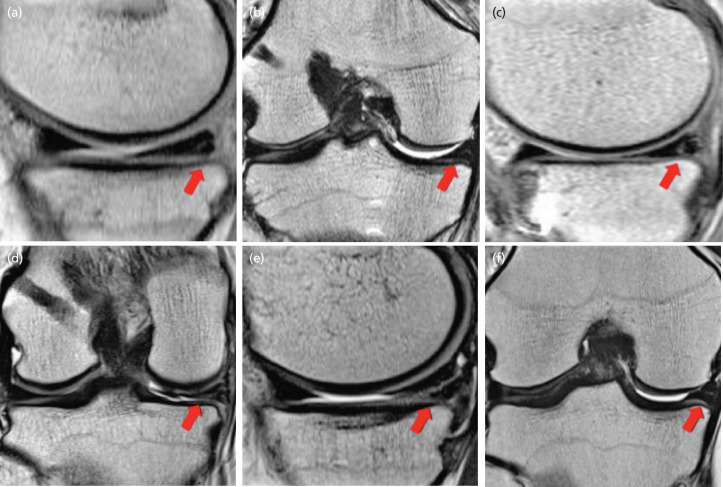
Crues’ grading system using magnetic resonance imaging (a, b) Grade 1 in sagittal and coronal view. No abnormal signal is seen on the meniscus. (c, d) Grade 2 in sagittal and coronal view. Red arrows indicate linear signal without communication with an articular surface. (e, f) Grade 3 in sagittal and coronal view. Red arrow indicates linear signal communicating with articular surface.
